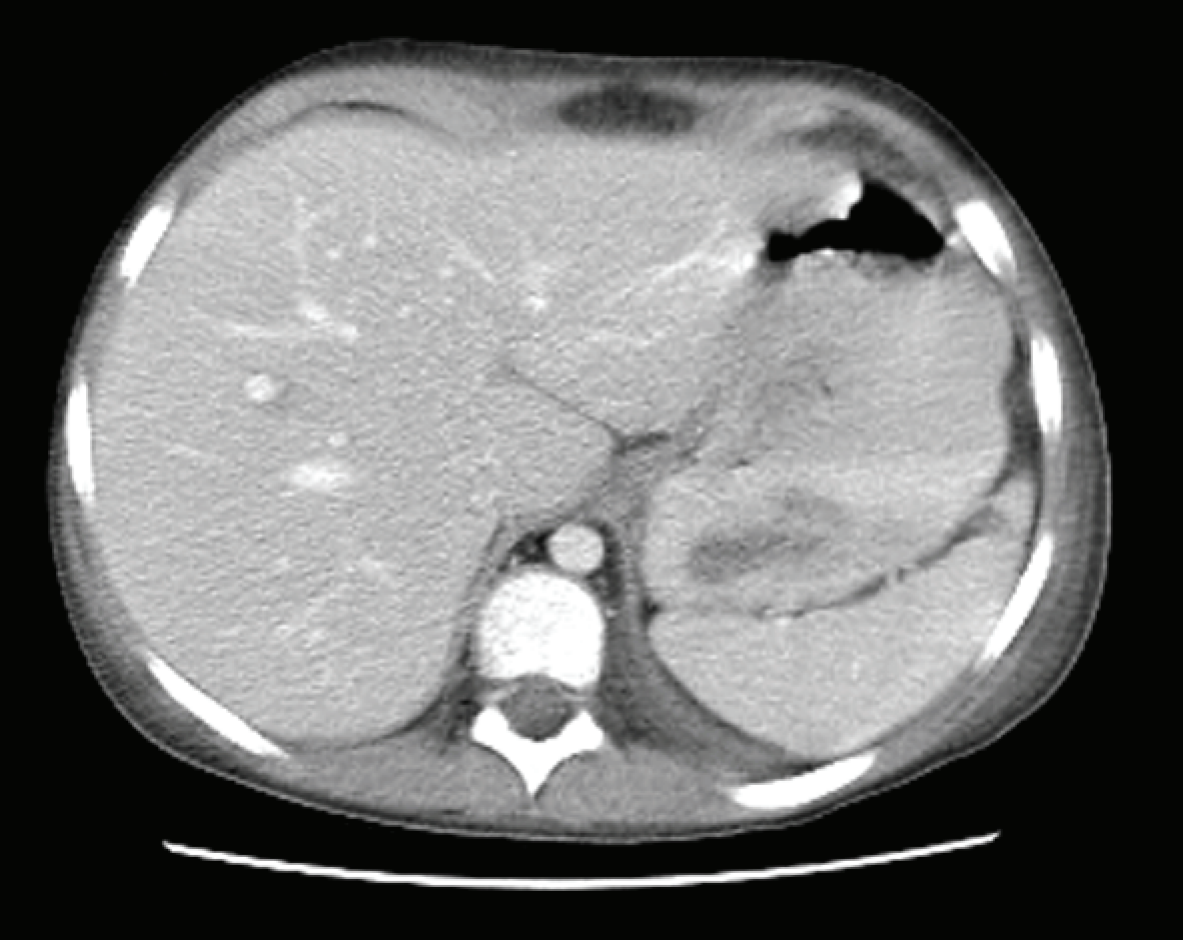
Computed tomography scan of the abdomen at diagnosis. Exophytic masses with multiple filling defects are present in the gastric mucosa.
